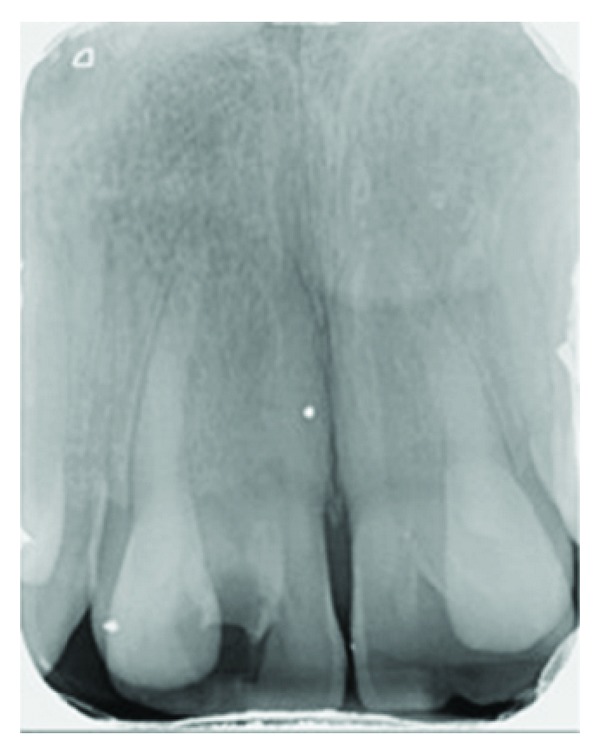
Periapical radiograph of the geminated incisors before treatment.
